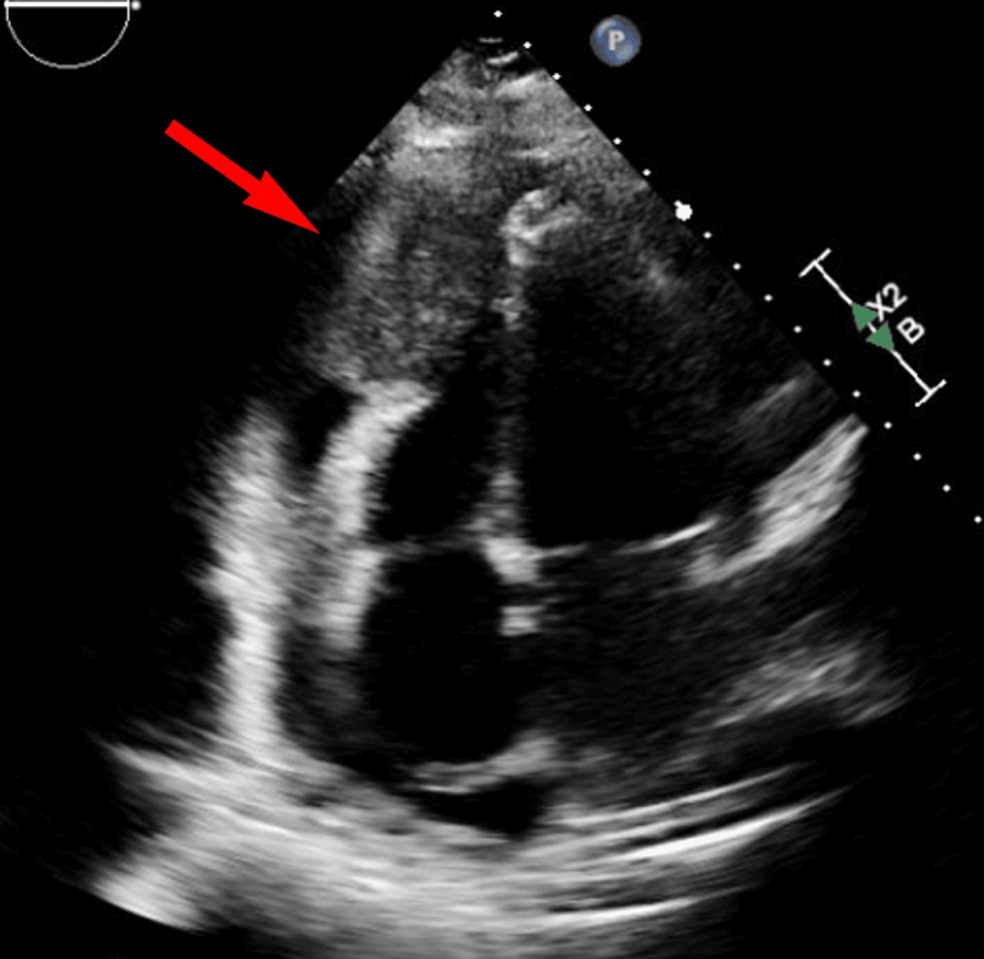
Transthoracic echocardiogram apical four-chamber view Transthoracic echocardiogram apical four-chamber view showed a heterogenous irregularly shaped mass adjacent to the right ventricle apex.
